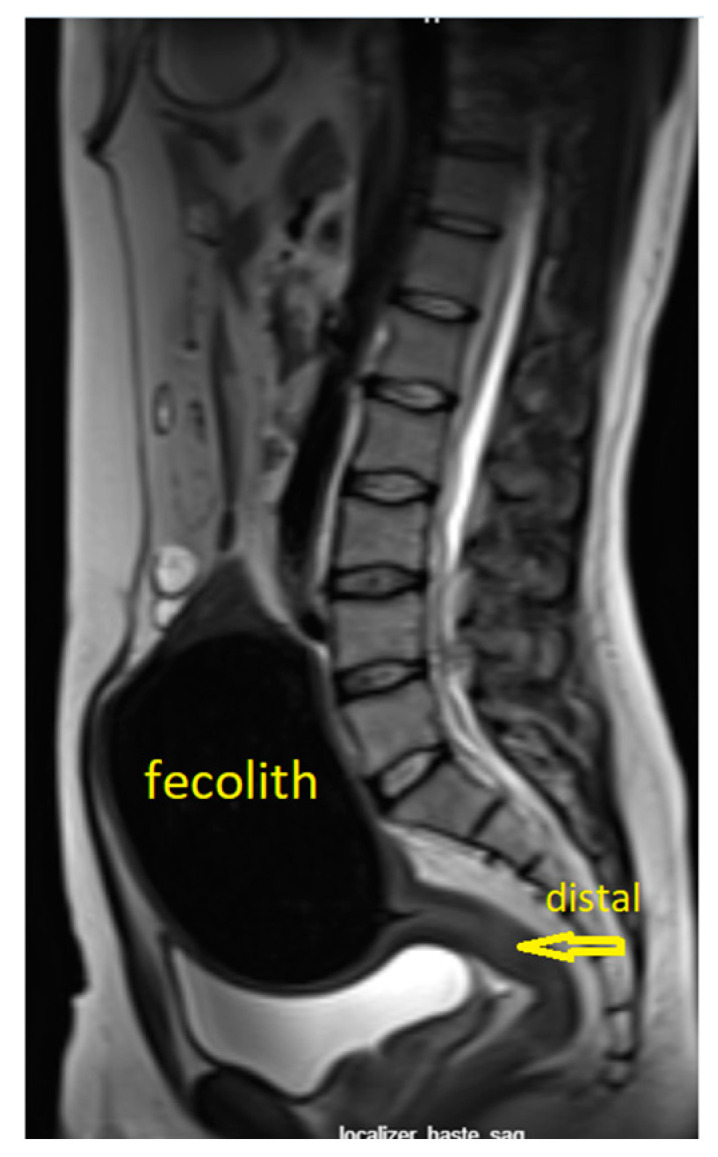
Magnetic Resonance Imaging enterography with large fecal mass at sigmoid colon.
